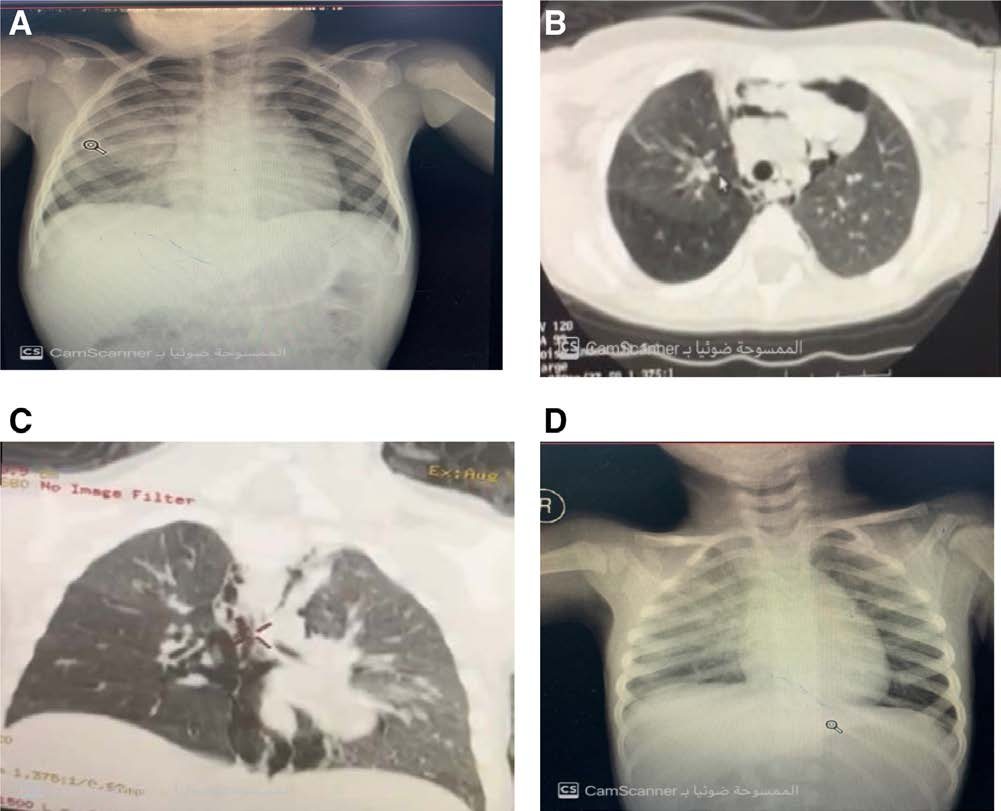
Chest radiography and CT scan were performed for case 2 before, during, and after FB removal. (A) AP chest radiograph upon patient’s arrival to the ER. (B) Axial view of the chest CT with pneumomediastinum. (C) Coronal view of the chest CT with pneumomediastinum. (D) Postoperative chest radiograph AP view. CT = computed tomography.
